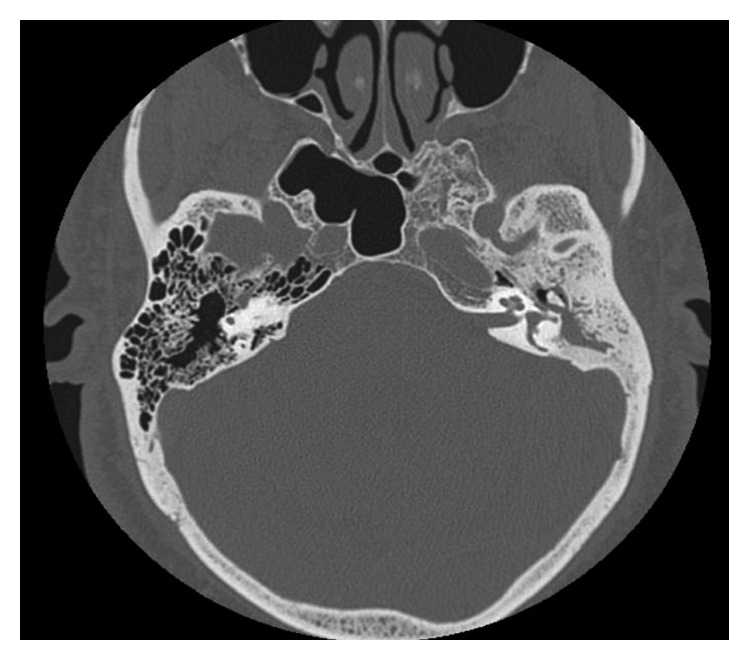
High-resolution CT scan (axial cut) of the temporal bone. Total opacification of the left-sided hypo- and mesotympanum by a soft tissue density. Mastoid air cells totally opacified with resorption of septae and partially sclerosed bone. Partial dehiscence of the mastoid portion of the facial nerve canal. The left tympanic membrane is thickened and retracted inwards. The left middle ear ossicles and scutum are intact. Normal aeration of the external auditory canals with intact bony and cartilaginous boundaries. The right middle ear cavity is normally aerated with intact ossicles. The right mastoid air cells are unremarkable.
